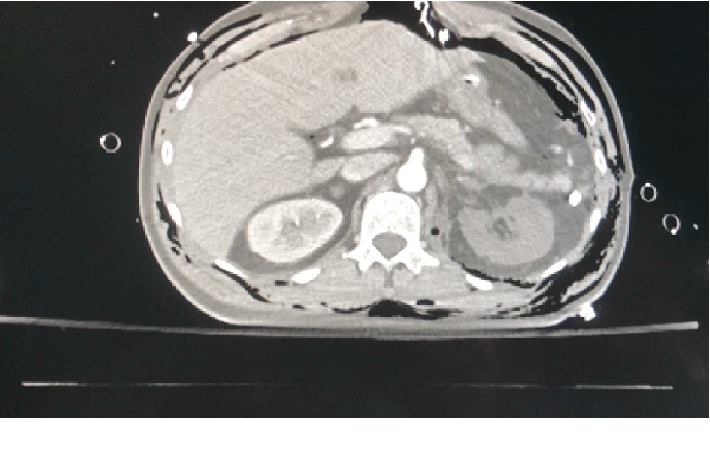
CT scan with contrast showing nonenhancing left kidney consistent with left renal artery injury.
